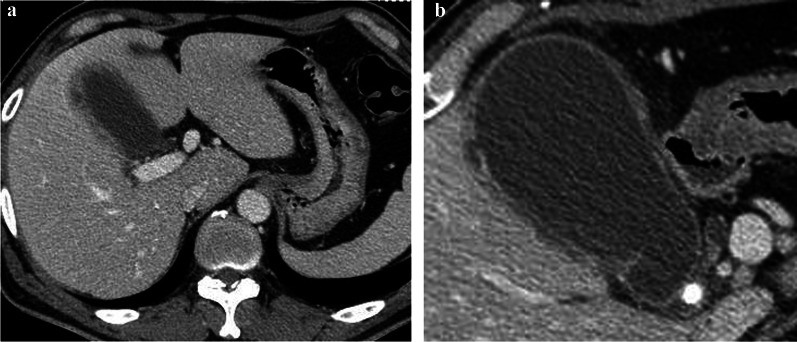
a CT showing thickening of the gallbladder wall. b Gallbladder stone impacted at the cystic duct
